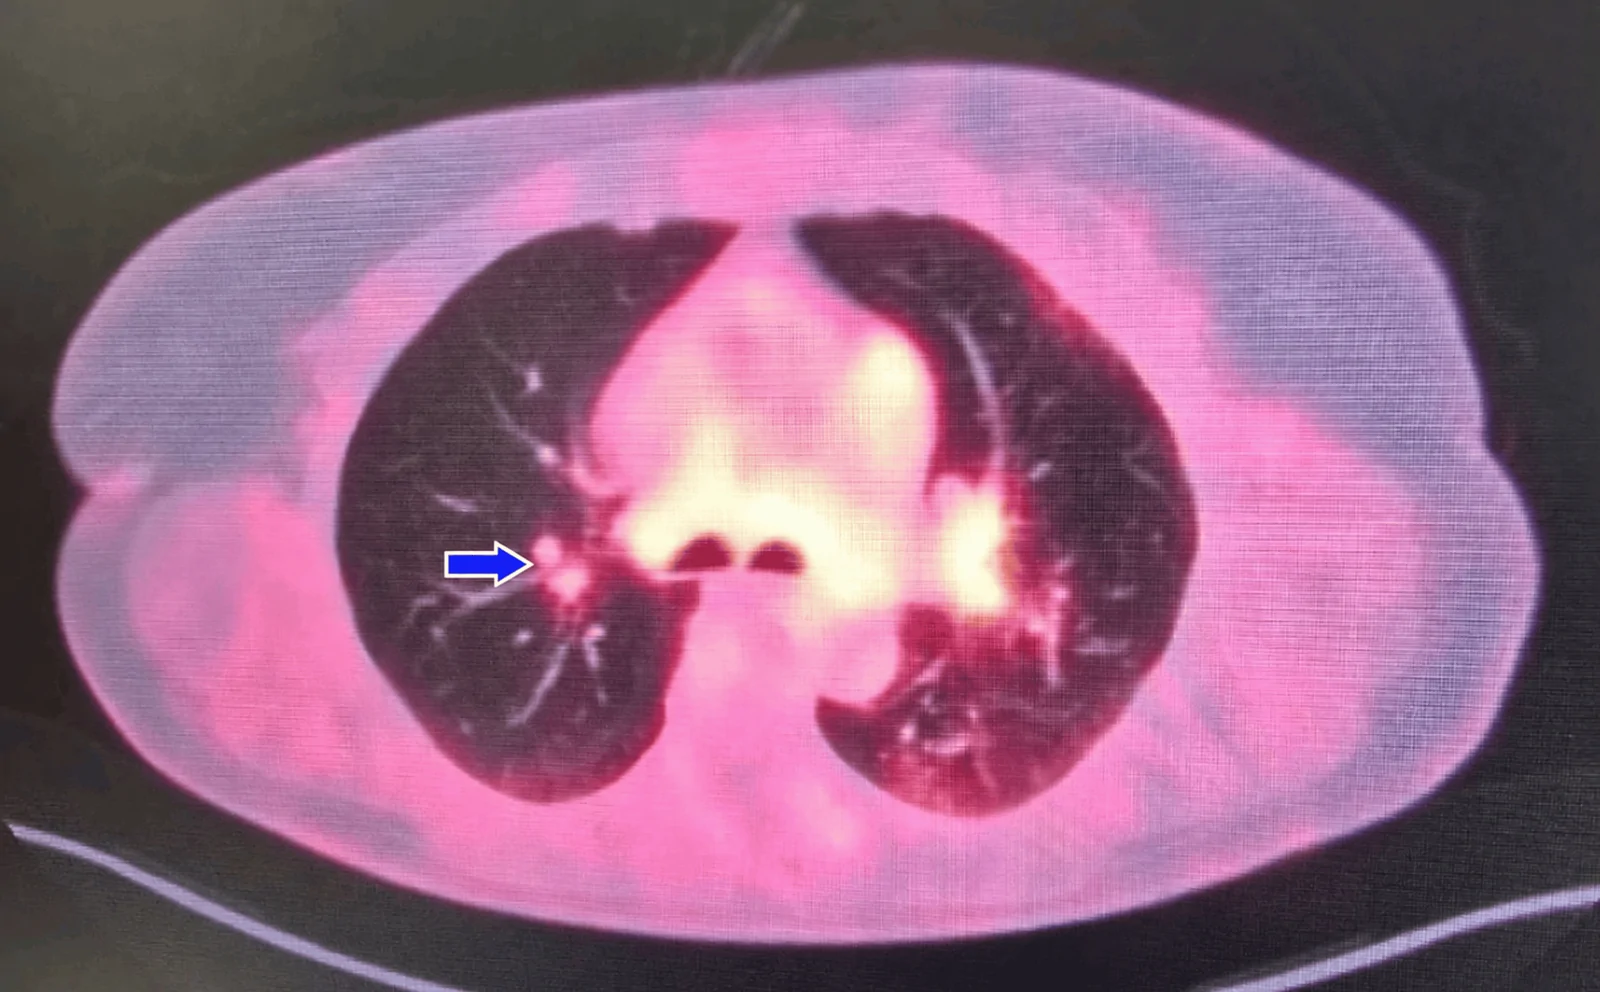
Preoperative axial fused 18F-FDG PET/CT image Axial ^18^F-FDG PET/CT image demonstrating increased metabolic activity in the bilateral hilar and mediastinal lymph nodes. The blue arrow indicates an FDG-avid right hilar lymph node. ^18^F-FDG PET/CT: fluorine-18 fluorodeoxyglucose positron emission tomography/computed tomography
